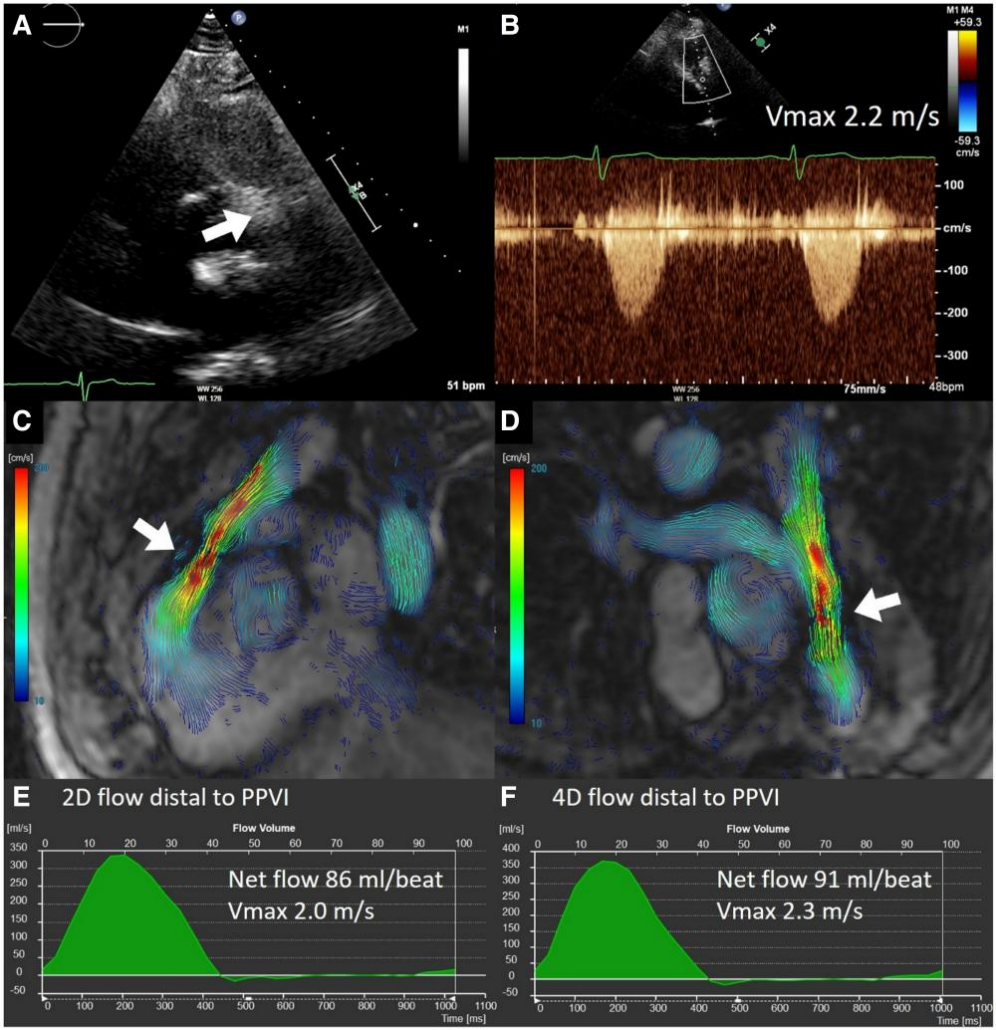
Example of a transthoracic echocardiogram and cardiovascular magnetic resonance in a patient after implantation of a percutaneous pulmonary valve implantation. (*A*) parasternal view of the right ventricular outflow tract with the transcatheter pulmonary valve in situ (Melody valve, white arrow), (*B*) continuous wave Doppler signal with maximal velocity of 2.2 m/s. (*C* and *D*) 4D flow cardiovascular magnetic resonance images of the right ventricular outflow tract and pulmonary artery and branches. (*E* and *F*) corresponding 2D and 4D flow measurements distal to the valve. The maximum velocity was 2.0 and 2.3 m/s, respectively, with a net flow of 86 and 91 mL/beat without any regurgitation.
